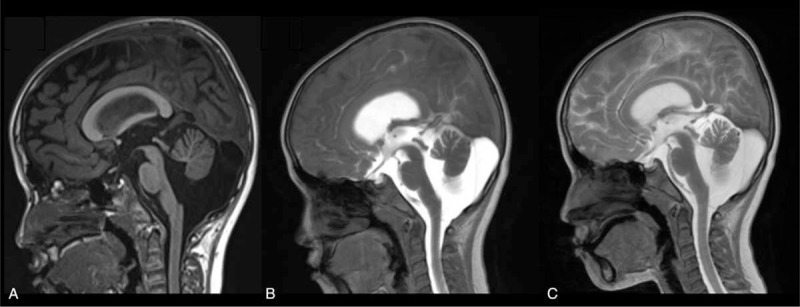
AC in the cistern magna of an 11-year-old male. An arachnoid cyst located in the cistern magna was unexpectedly found during CT after a head injury. The cyst did not cause any symptoms. One hour after cisternography, the cyst was enhanced in the sagittal T1 sequence (B). Sagittal T1 at 24 hours showed the signal inside the cyst was equal to the surrounding cistern, indicating a complete communicating AC (C). AC = arachnoid cyst, CT = computed tomography.
